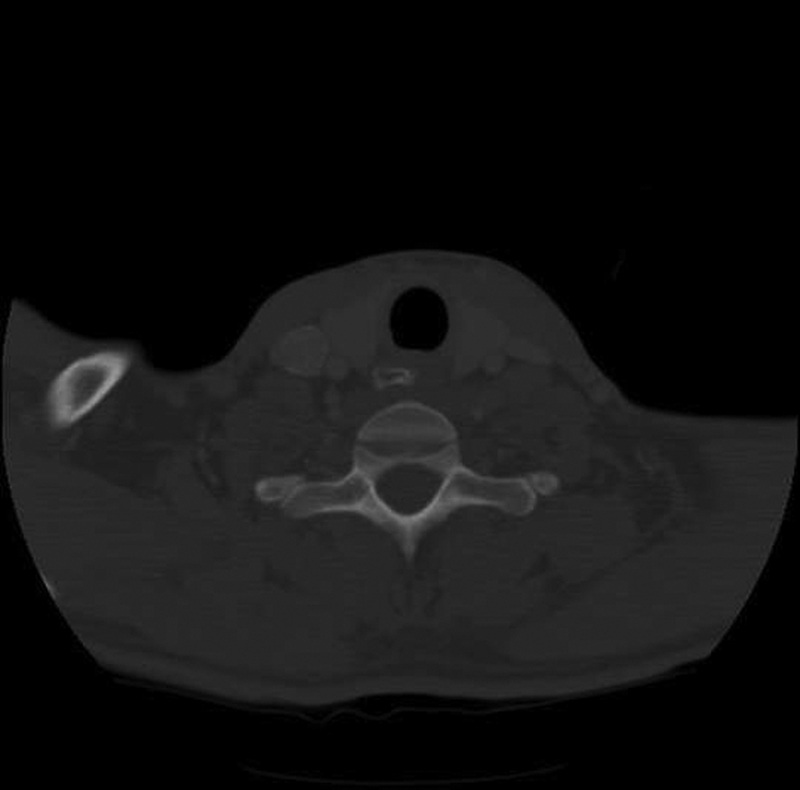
Cervical computed tomography shows a chicken bone in the first narrowing of the esophagus.
